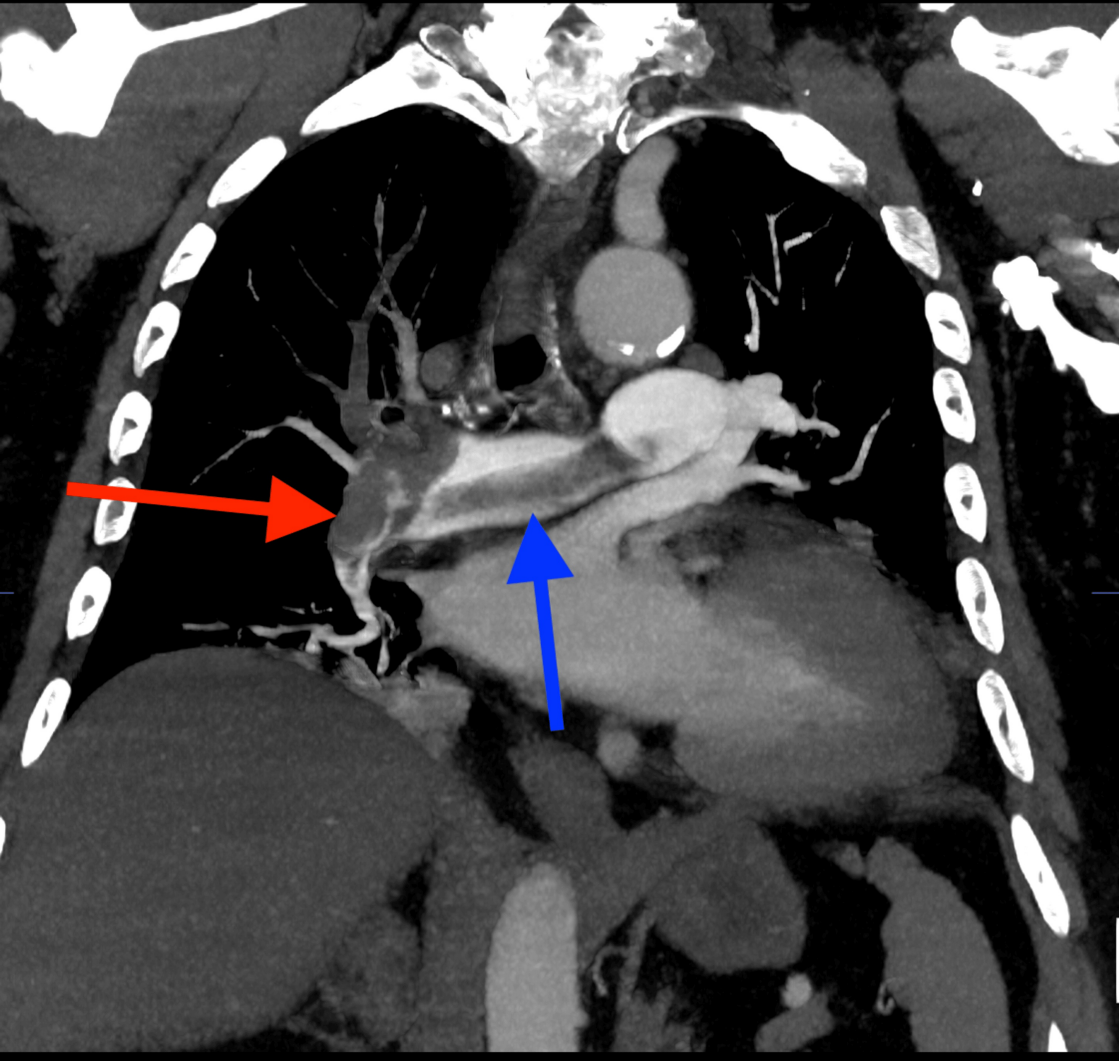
Coronal reconstruction of CT pulmonary angiogram shows (blue arrow) saddle thrombus and (red arrow) thrombus in the right pulmonary artery extending into lobar branches.
